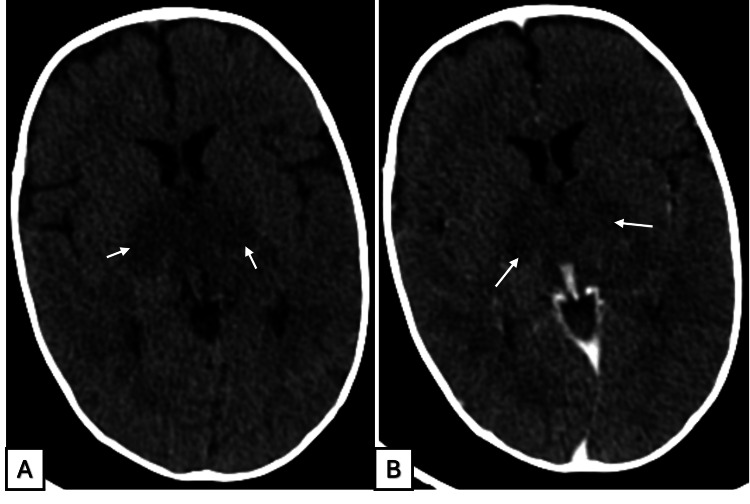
Brain CT findings in ANEC A CT scan without (A) and with contrast enhancement (B) shows bithalamic ill-defined areas of hypodensity, with no visible enhancement. ANEC, acute necrotizing encephalopathy of childhood
